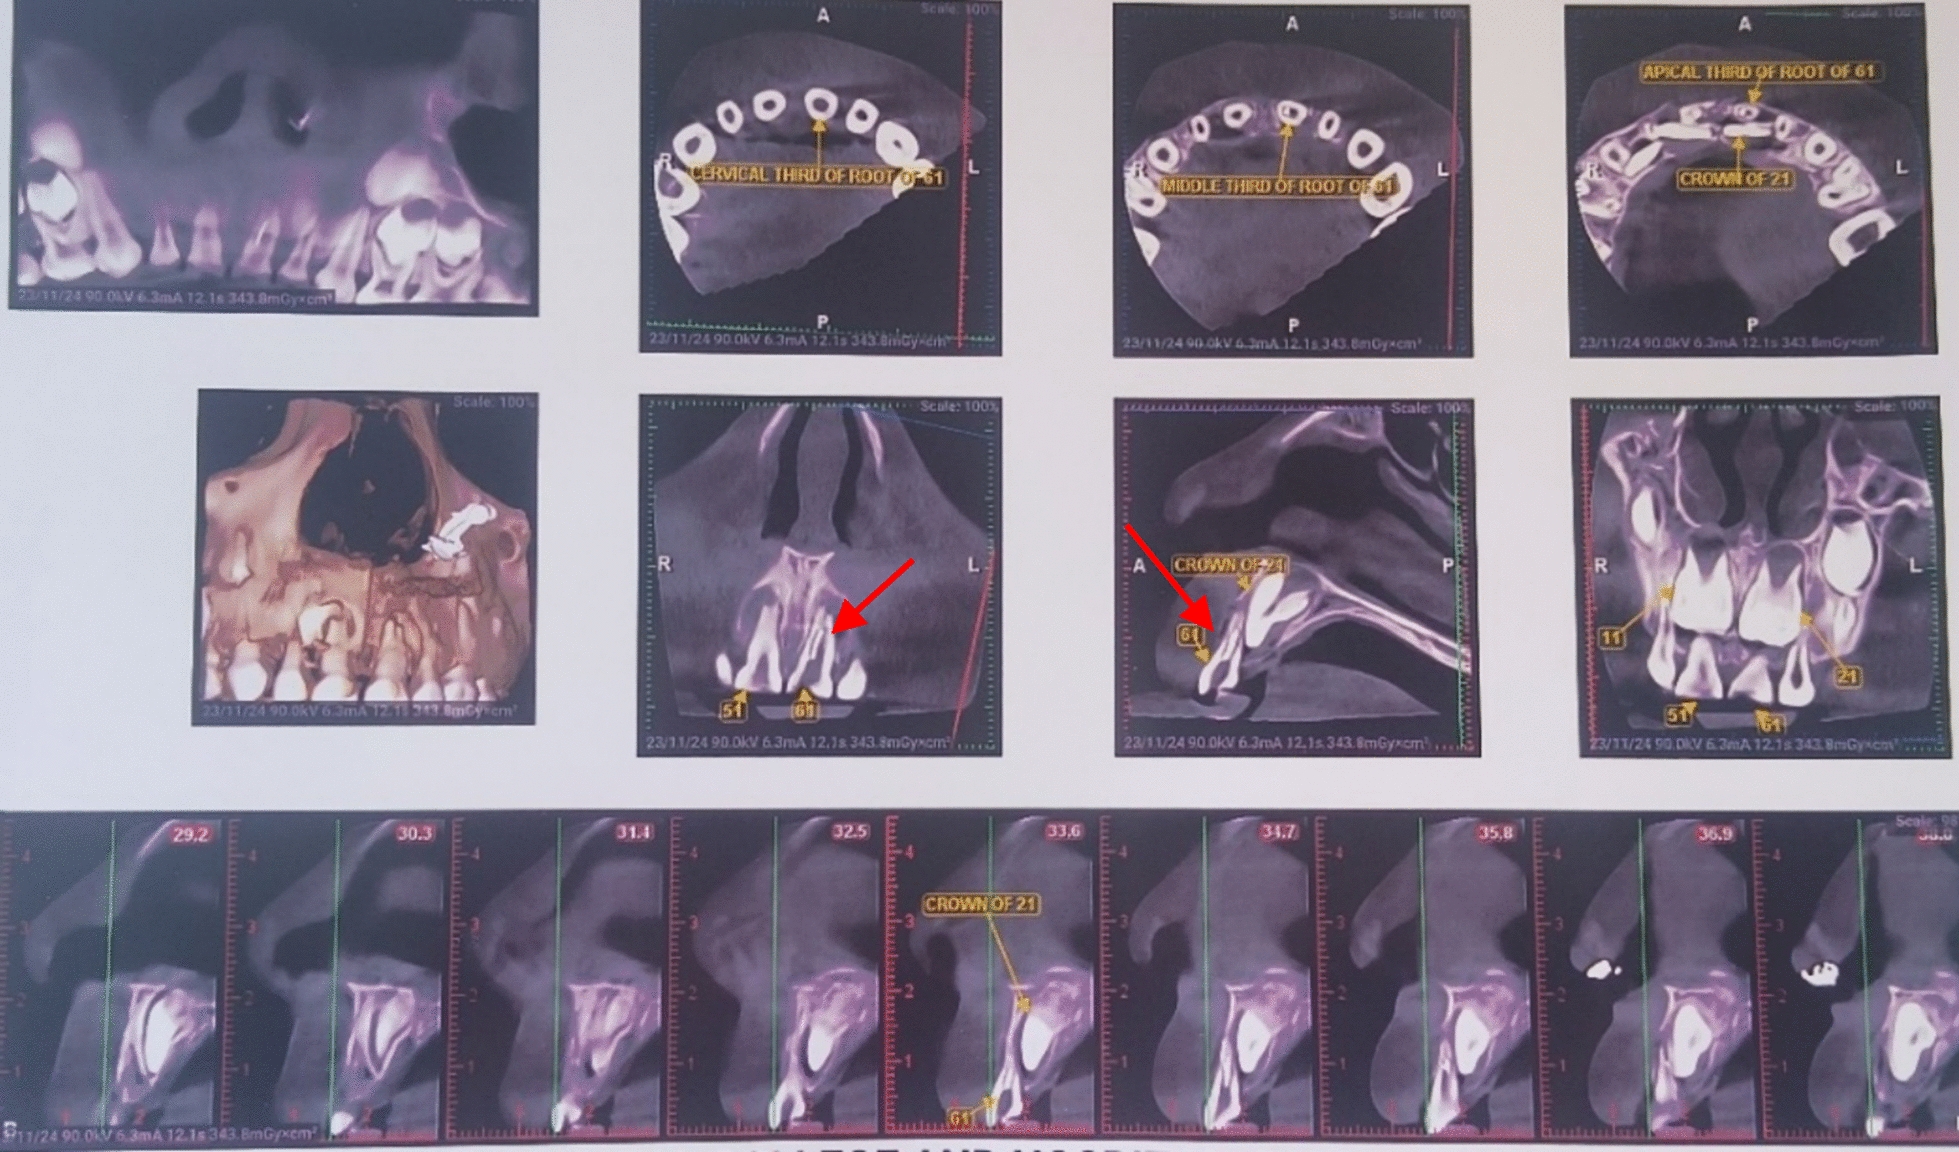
Cone beam computed tomography of tooth 61 with red arrow confirming the location of foreign object in the primary tooth
